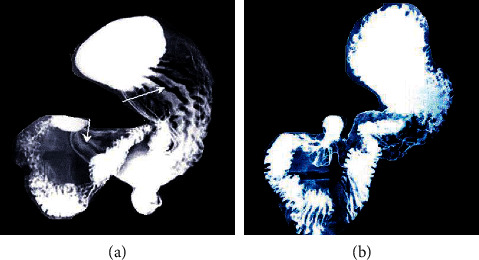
Results of gastrointestinal X-ray imaging. (a) Normal X-ray of gastrointestinal tract. (b) Positive gastrointestinal changes in HP.
